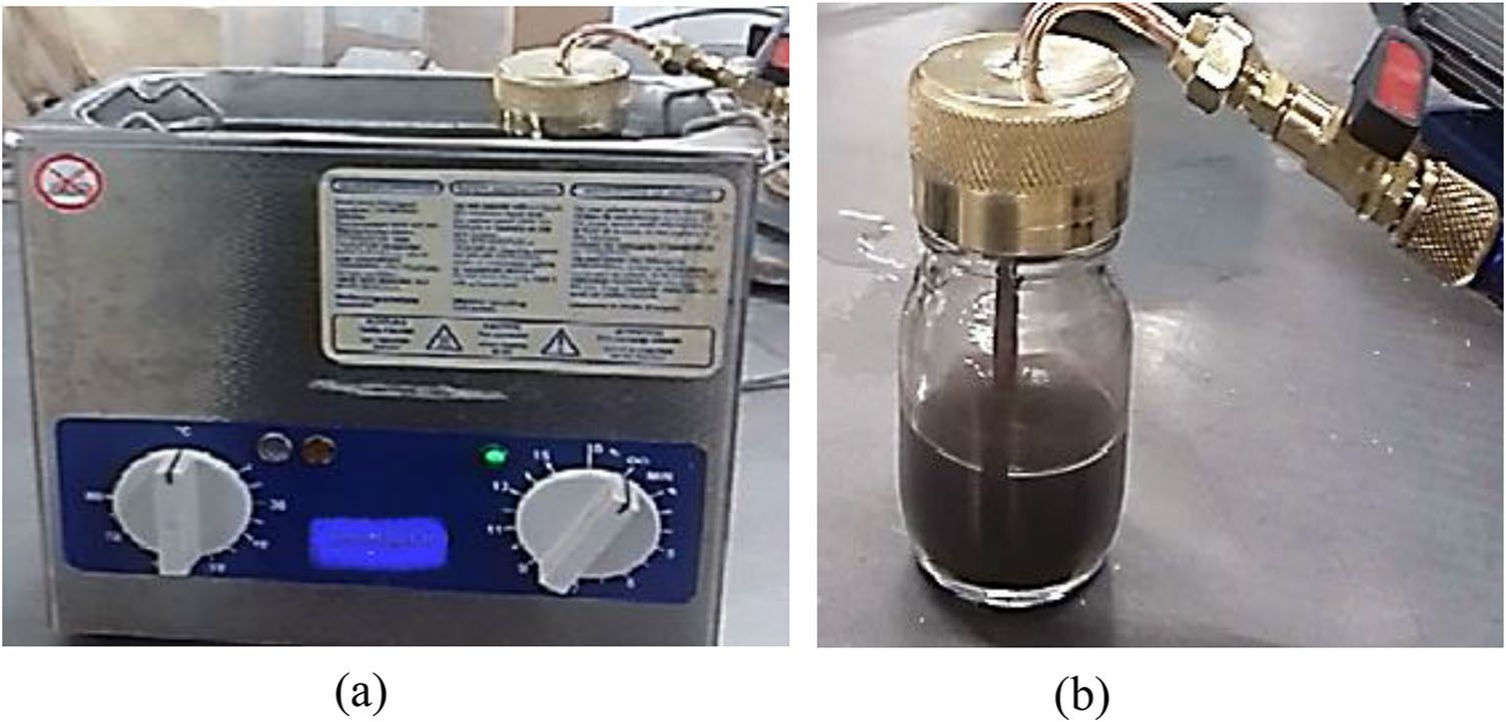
(a) Ultrasonic device, and (b) the mixing vessel of the nanorefrigerant.
